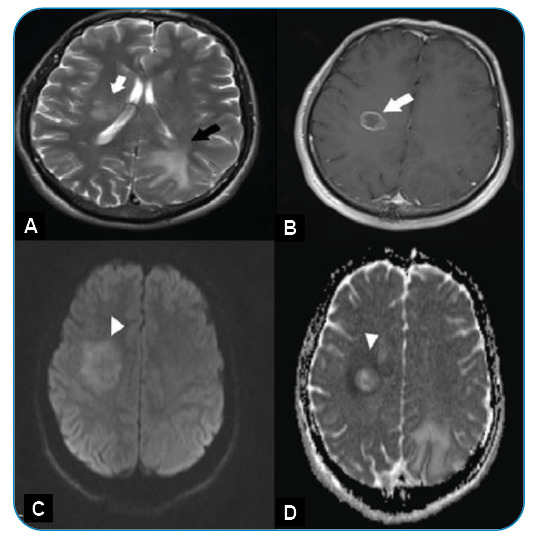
(A) The axial T2-weighted image demonstrates a heterogeneous lesion with indistinct borders in the right frontal lobe (white arrow) and vasogenic edema in the left parieto-occipital white matter without a mass (black arrow). (B) The contrast-enhanced axial T1-weighted image shows mild peripheral contrast enhancement (white arrow). (C,D) The diffusion-weighted image and apparent diffusion coefficient map reveal peripheral restricted diffusion (arrow head).
